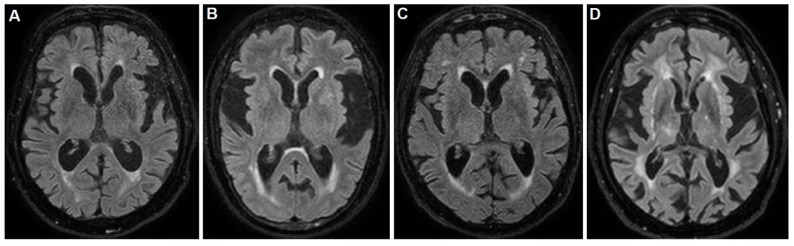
Brain magnetic resonance imaging (MRI) short tau inversion recovery (STIR) sequences of (A) patient 1: note the enlargement of the ventricular system and subarachnoid spaces with mild hyperintensity of the occipital horns periventricular white matter; (B) patient 2: note the enlargement of subarachnoid spaces with diffuse deepening of the cortical sulci and a thinning of the cortical mantle, especially at the fronto-temporal lobes. The hyperintensity of the occipital horns periventricular white matter is also visible; (C) patient 3: note the slight diffuse shrinkage of the subcortical grey matter with several hyperintense lesions at semioval centers; (D) patient 4: note the subcortical chronic ischemic lesions with vascular encephalopathy and periventricular diffuse hyperintensity. Note the atrophy of insula as a common feature of these brains.
